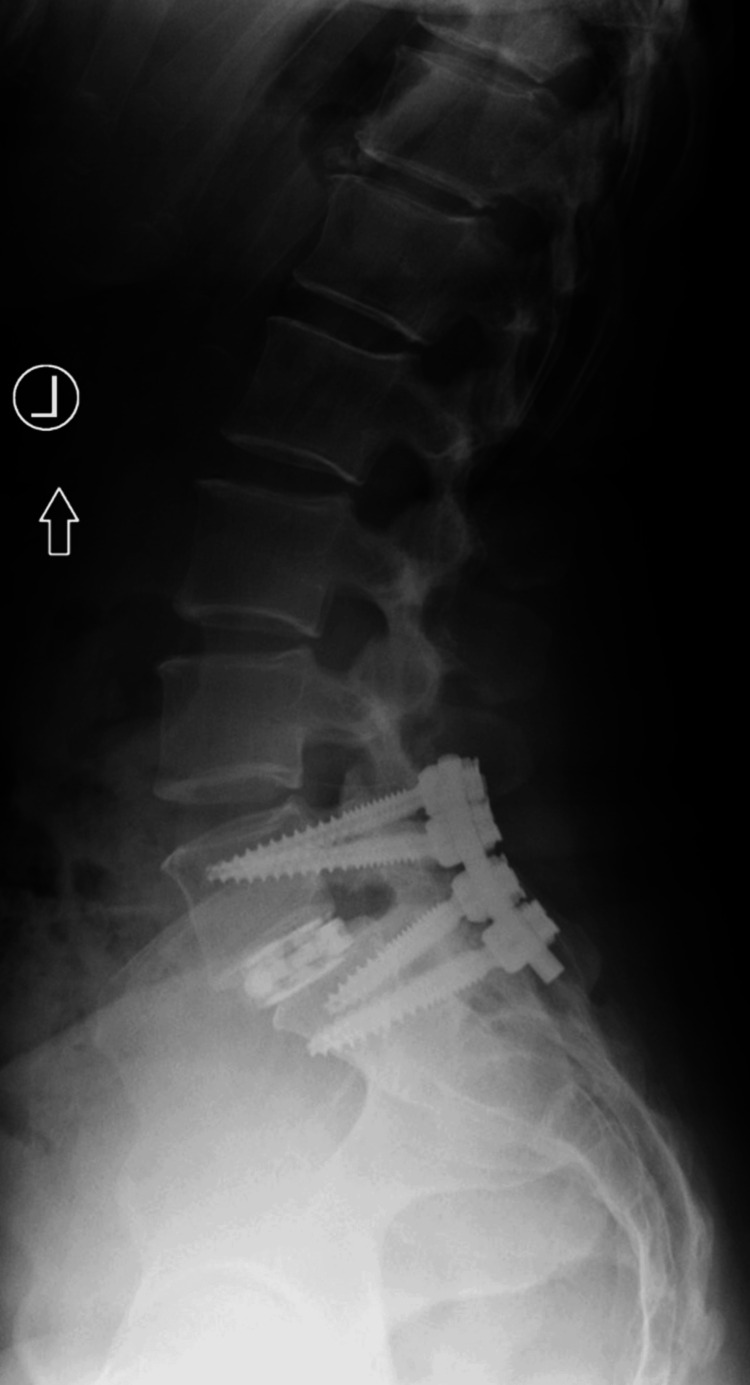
Post-operative lateral radiograph for Case 1.
